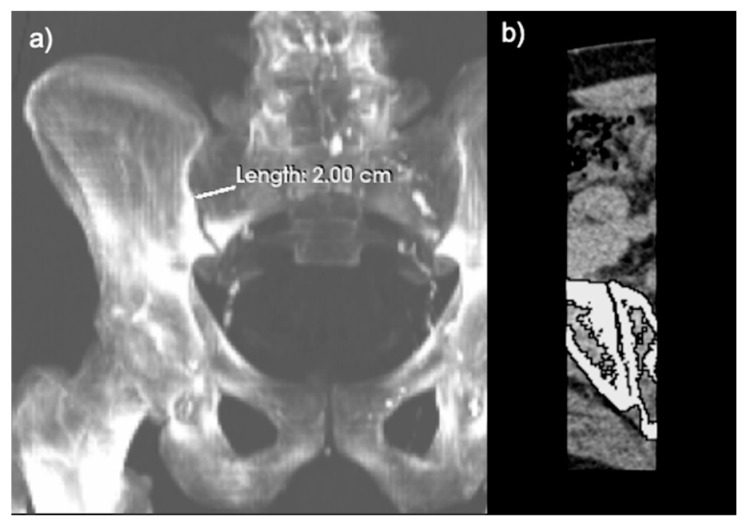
Delineation of the region of interest (ROI) of the sacroiliac (SI) joint. (a) Coronal view of the CT segmentation showing the lateral and medial borders forming 2 cm from the articulation of the sacrum and ilium. (b) Axial view depicting the process of morphological closing.
